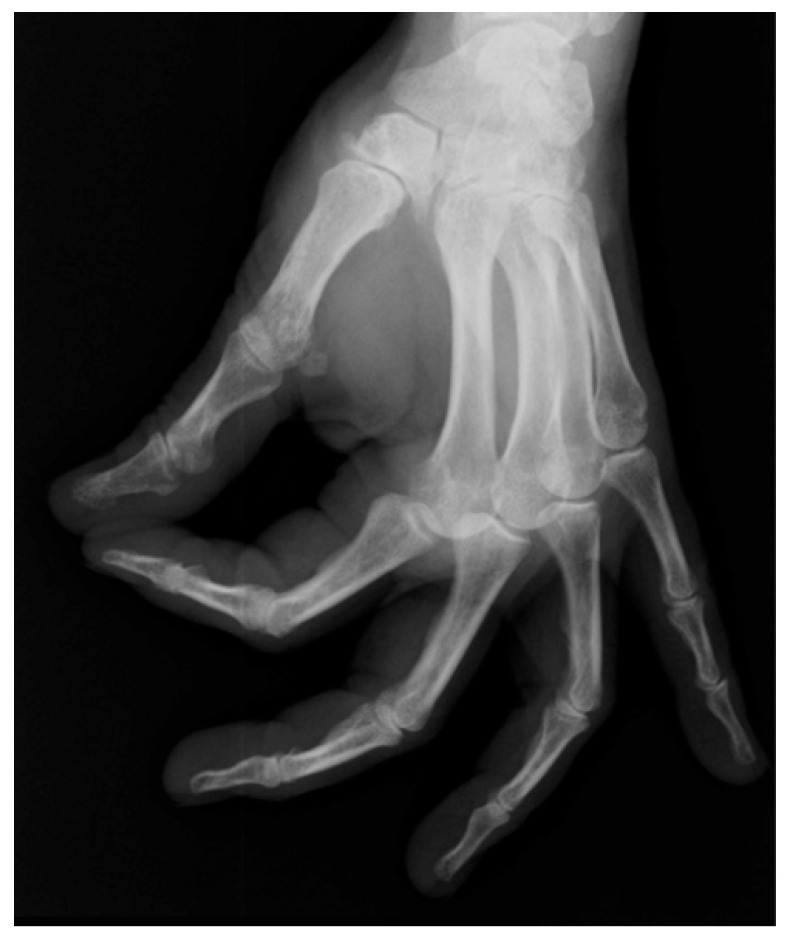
Radiographic image showing rhizarthrosis on a hand X-ray.
